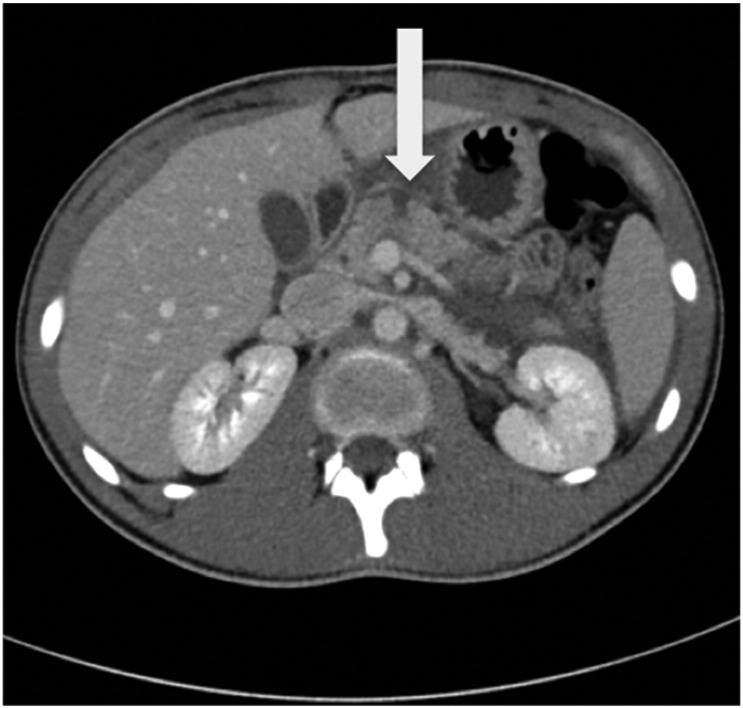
Initial abdominal CT 24 h post-injury showing transection of the neck of the pancreas consistent with AAST-OIS Grade IV injury.
